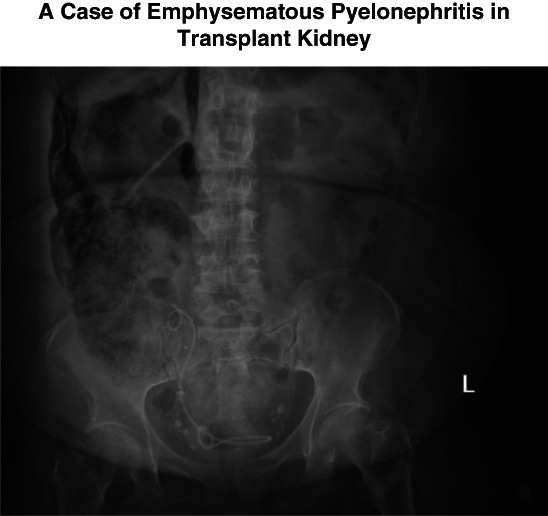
X-ray of the abdomen erect at initial presentation demonstrates significant amount of retroperitoneal free air seen along the right flank and right paraspinal region and diffuse emphysematous changes involving the right transplant kidney.
